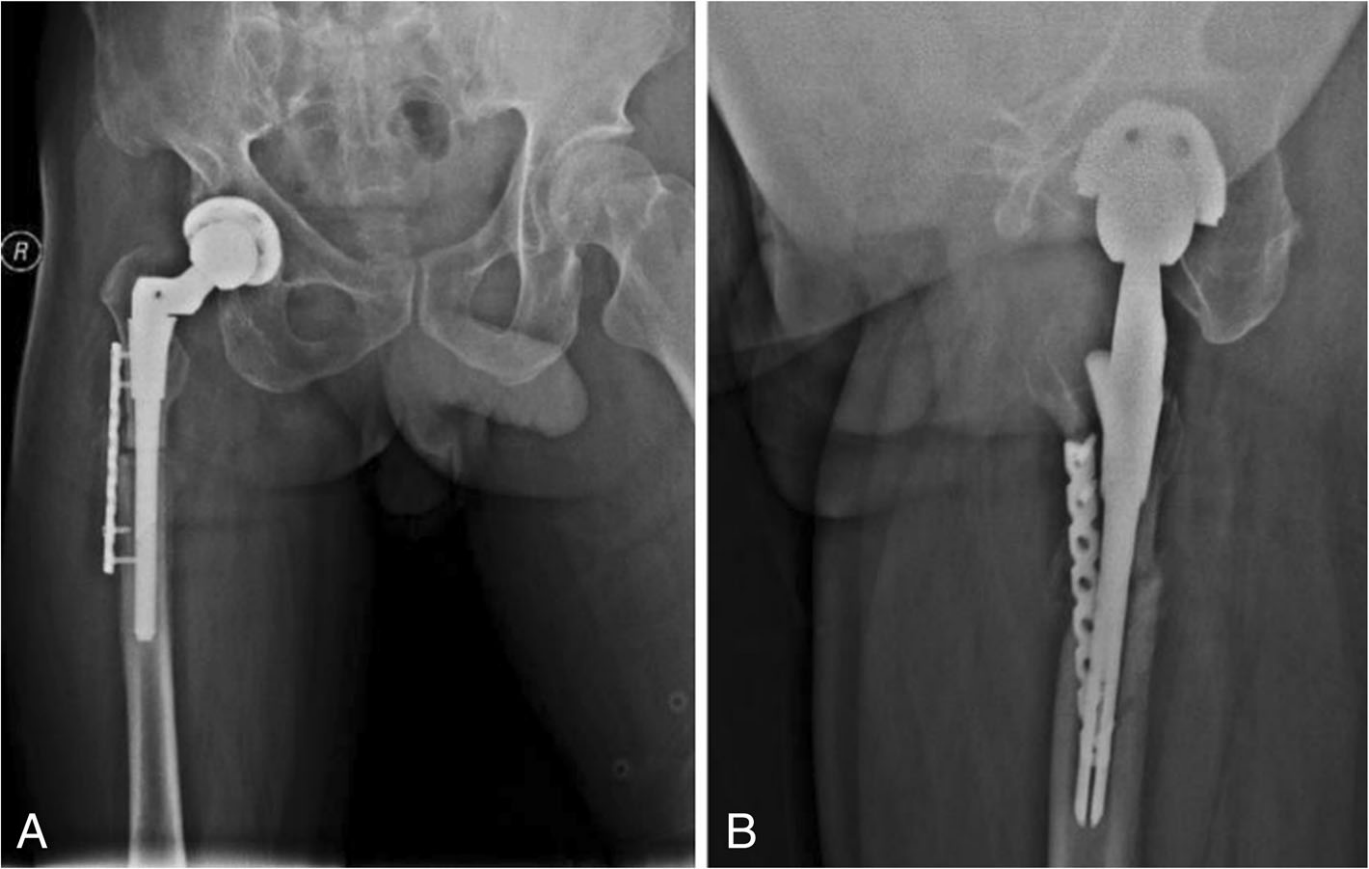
Radiographs of the right hip at two and a half months after internal fixation was applied. (a) anteroposterior view of the right hip, (b) lateral view of the right hip
